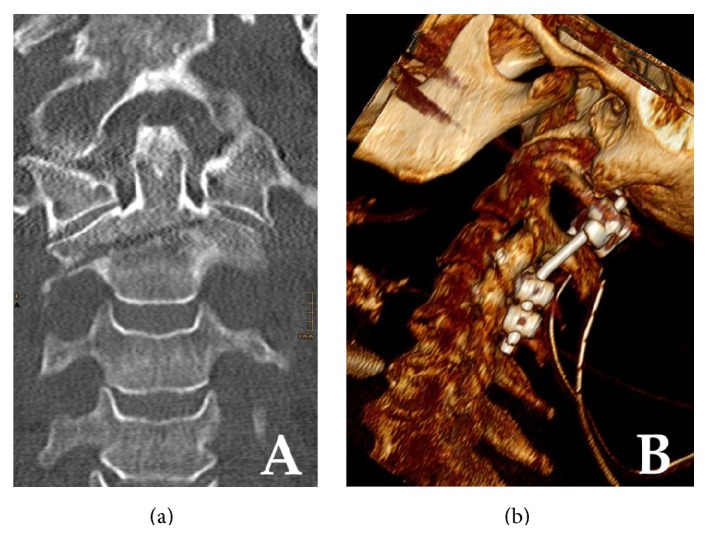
Cervical spine CT imaging of a 71-year-old woman. (a) Coronal plane CT imaging showing the minimally displaced oblique type axis body fracture. Note the marked similarity of the fracture pattern when compared to that shown in Figure 1(a). (b) Three-dimensional computed tomographic VRT reconstruction following C1–C3/C4 posterior fusion with a polyaxial screw-rod construct.
